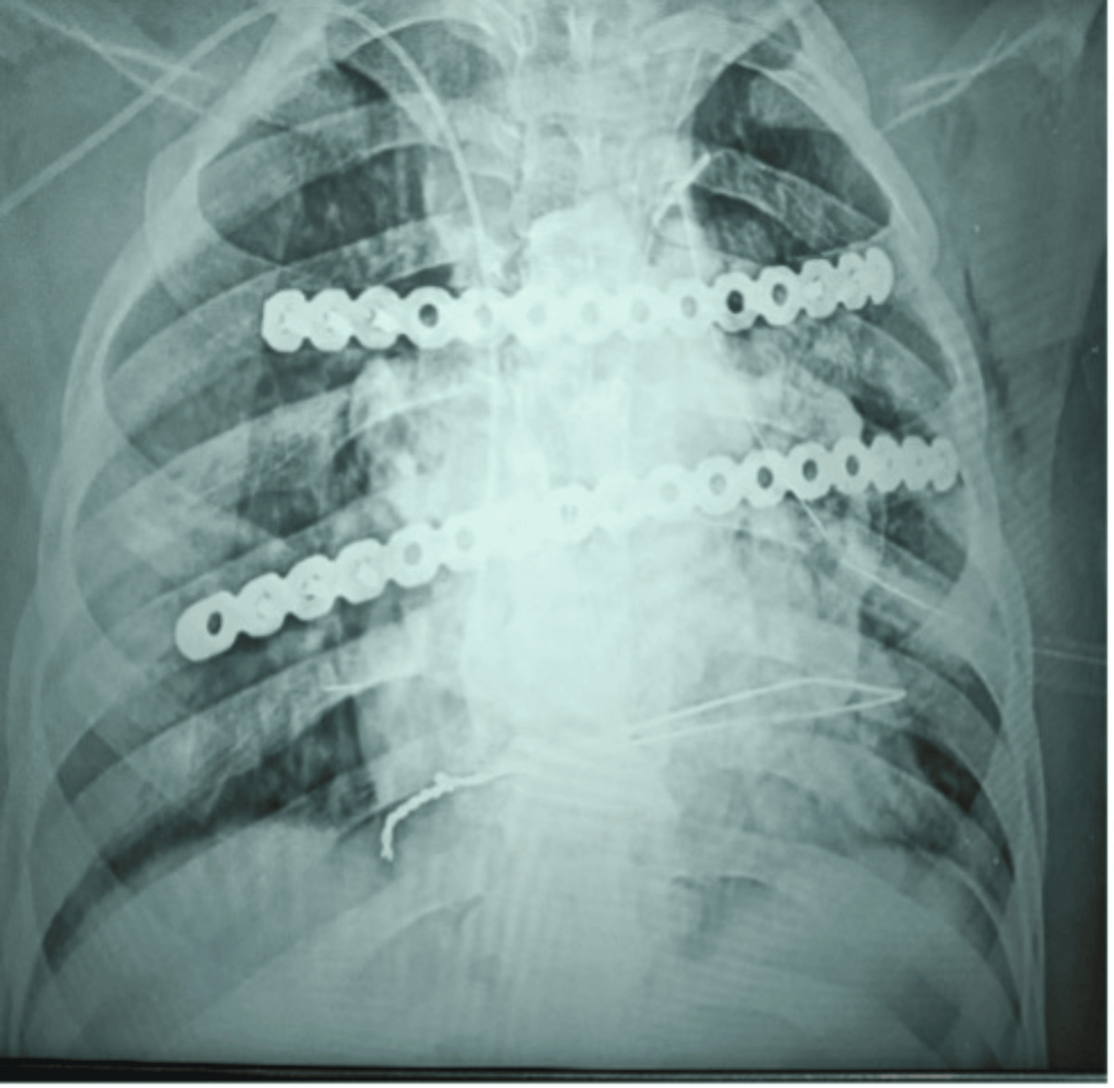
Immediate postoperative chest X-ray showing titanium plate fixation of neo-sternum and ribs Frontal chest radiograph revealing the position of the reconstructed sternum secured with dual horizontal titanium plates.Rib reconstruction and implant alignment are maintained.
